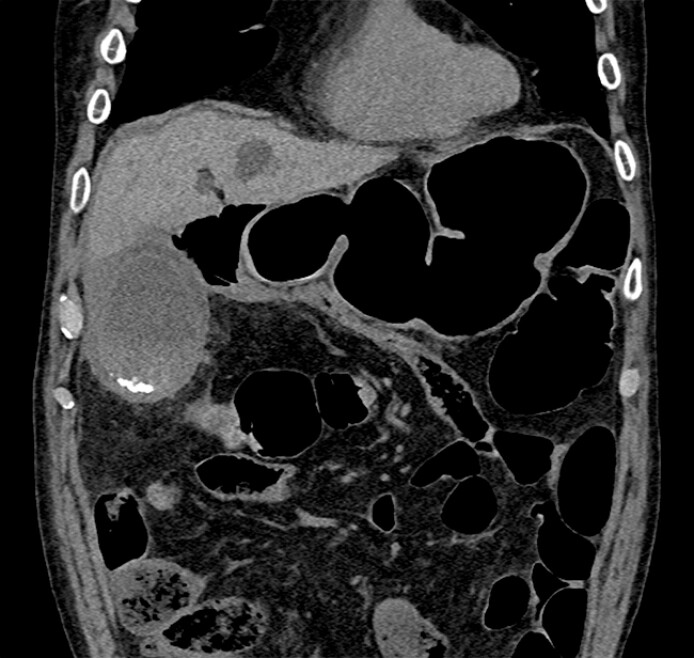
Computed tomography image showing acute cholecystitis on the patient’s day of admission.
